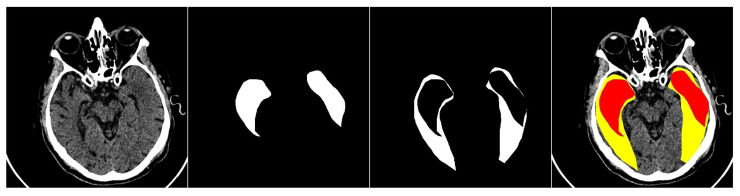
An example of an input image with three classes. The first one is the original CT scan, the second is the ischemic core mask, the third is the penumbra area mask, and the fourth is the visualization with mask overlay (ischemic core in red, penumbra area in yellow).
